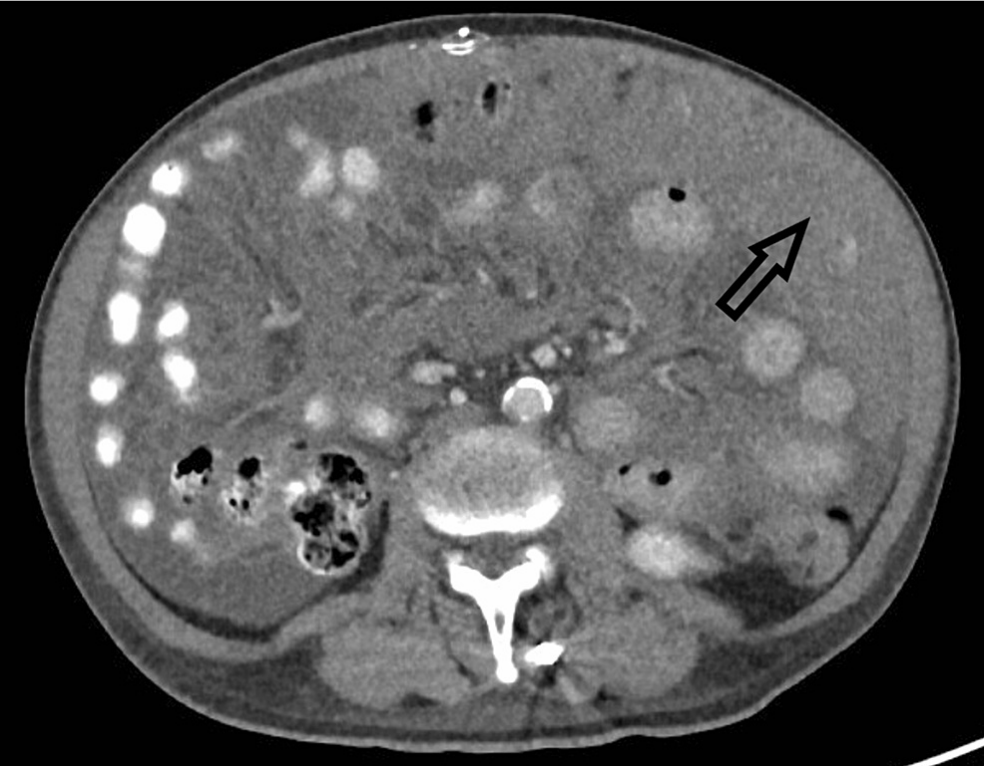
Contrasted abdominal CT showing diffuse omental thickening (indicated by arrow).
